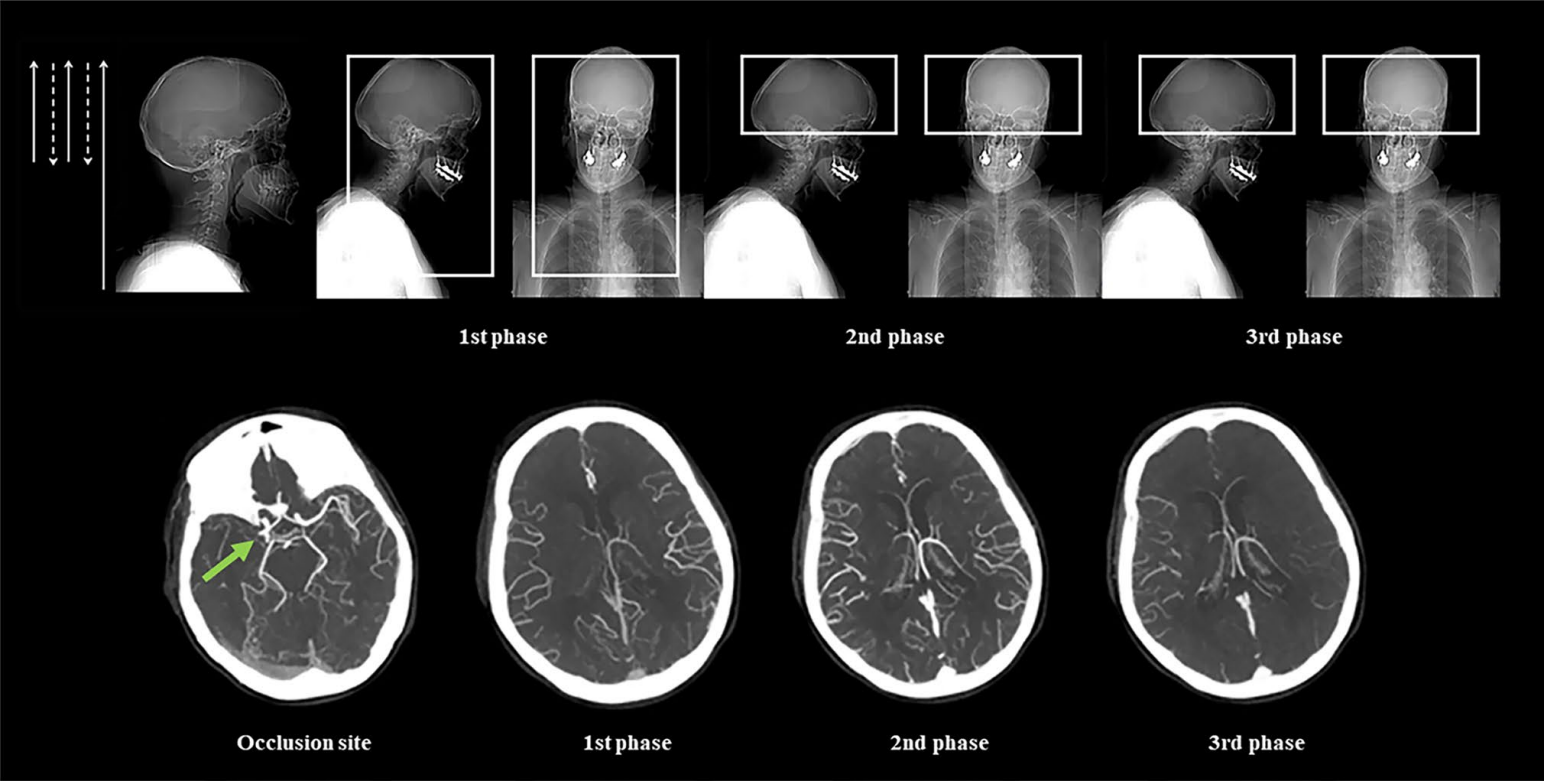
Multiphase CT angiography covering from the carotid bifurcation to vertex (first phase) and from the skull base to vertex (second and third phases) with maximum intensity projection (MIP) reconstructions for the visualization of cervical and intracranial vessels (first phase) and intracranial vessels only (second and third phases). Green arrow indicates occlusion site. CTA of the cervical and intracranial vessels was performed as follows: 0.7 mL/kg contrast (maximum 90 mL), 5- to 10-s delay from injection to scanning, 120 kV, 270 mA, 1 s/rotation, 0.625-mm-thick slices, and table speed 3.75 mm/rotation. The second phase was acquired after a delay of 8 s that allows for table repositioning to the skull base. Scanning duration for each additional phase was 3.4 s. Thus, the three phases were each 12 s apart. The axial images were reconstructed at 1-mm overlapping sections, and multiplanar reconstructions for axial, coronal, and sagittal images of the circle of Willis were performed with 3 mm thickness at 1-mm intervals. Thick-section axial maximum intensity projections at 24 mm thickness and 4-mm intervals were also reconstructed
